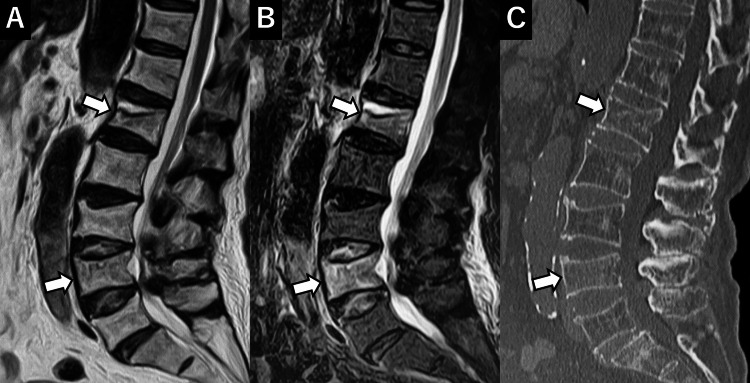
Initial MRI and CT A: T2-weighted image (T2WI), B: Short T1 inversion recovery (STIR), C: CT. New vertebral compression fractures are observed at L1 and L4 (arrows).
